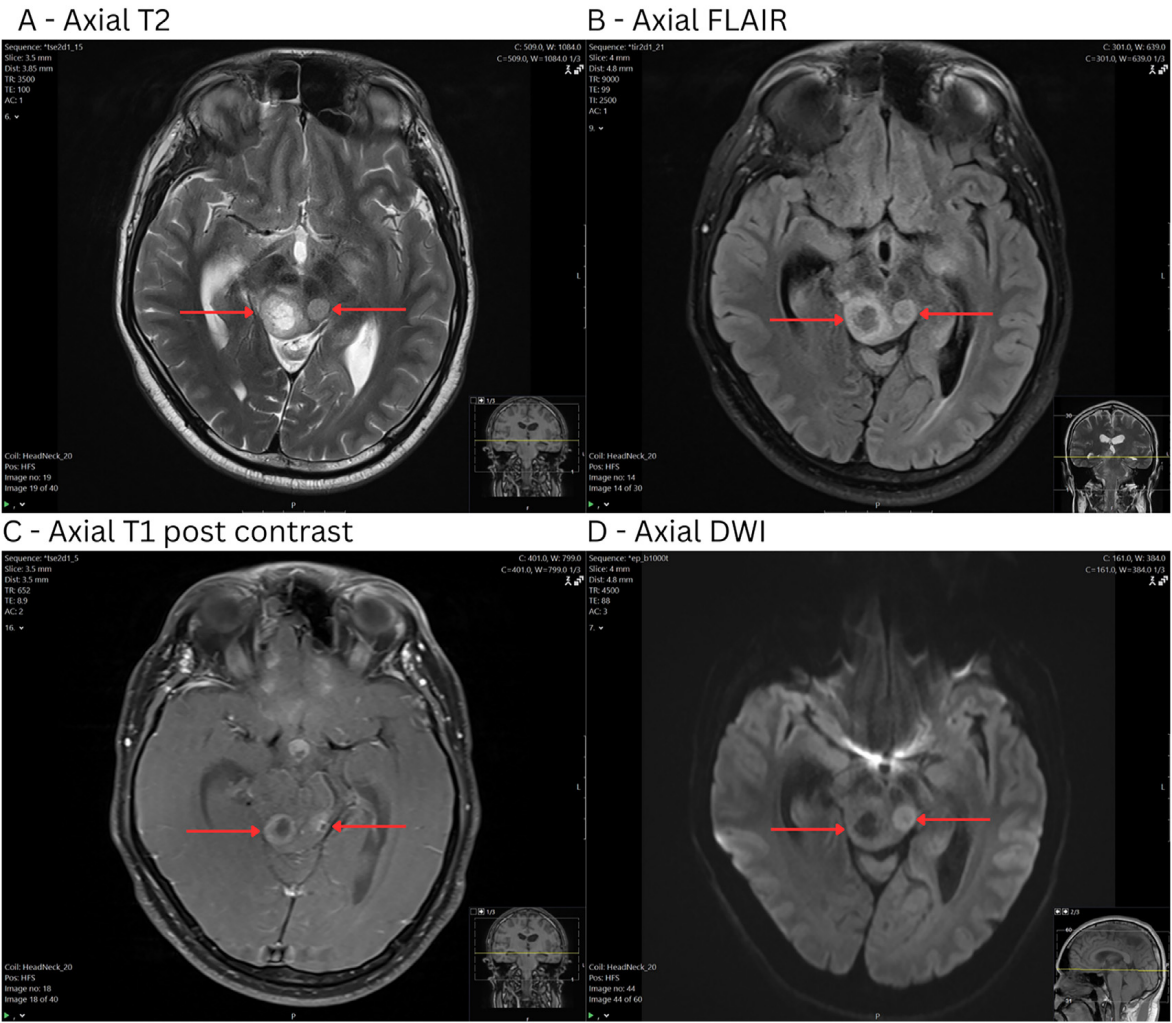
MRI images of midbrain lesions including: (A) Axial T2, (B) Axial FLAIR, (C) Axial T1 post contrast, (D) Axial diffusion-weighted images. 17 × 16 × 16 mm ovoid expansile mass in the right mid brain tectal plate, with a thick continuous peripheral rind of mildly T2 hyperintense, mildly T1 hypointense, avidly enhancing tissue with central nonenhancing, T2 hyperintense, FLAIR hypointense material. Small volume internal low SWI signal consistent with blood products based on the phase imaging. Immediately anteriorly adjacent similar but slightly less avidly enhancing 11 × 10 × 8 mm T2 hyperintense right posterocentral midbrain lesion with a probable small focus of central nonenhancement. Probable subtle diffusion restriction along the medial margin. Small volume internal low SWI signal consistent with blood products.
